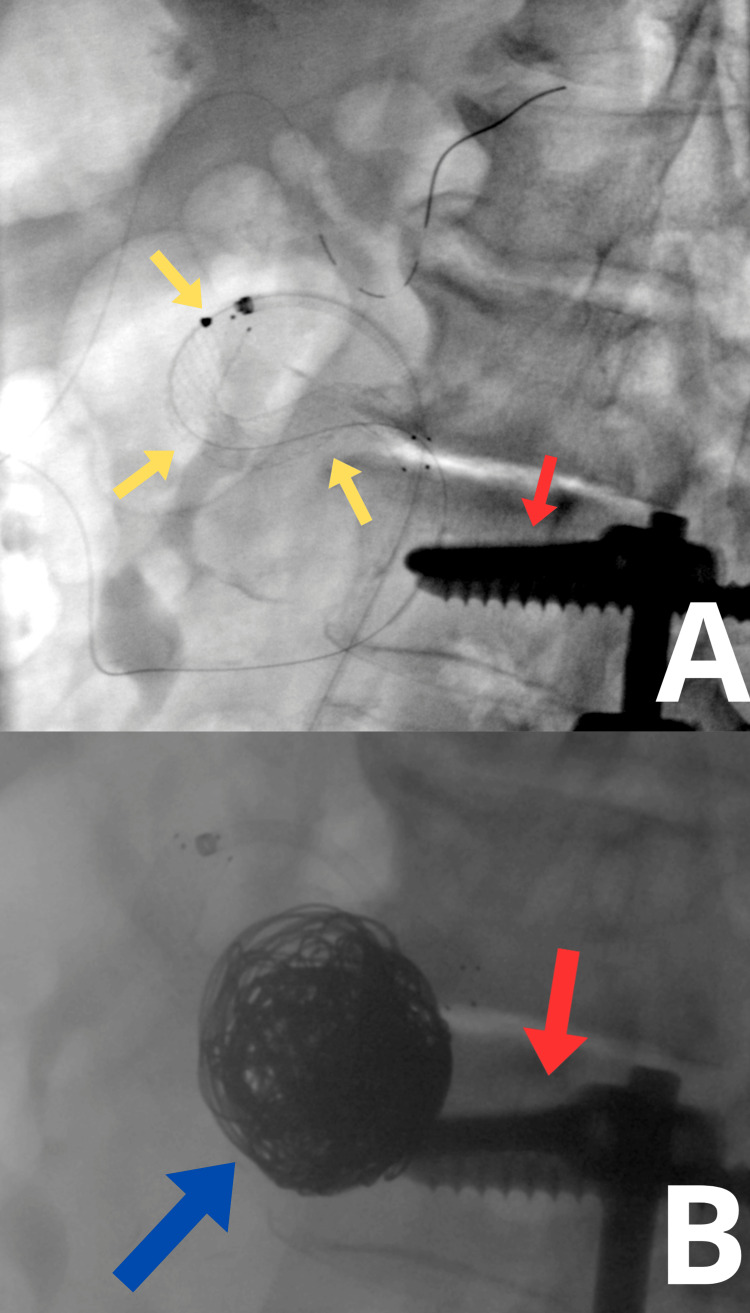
Intraoperative angio-CT. (A) Stent in the branch supplying blood to the liver. (B) Embolized aneurysm. The yellow arrow indicates the stent. The red arrow indicates spinal screws. The blue arrow indicates embolization coils.
